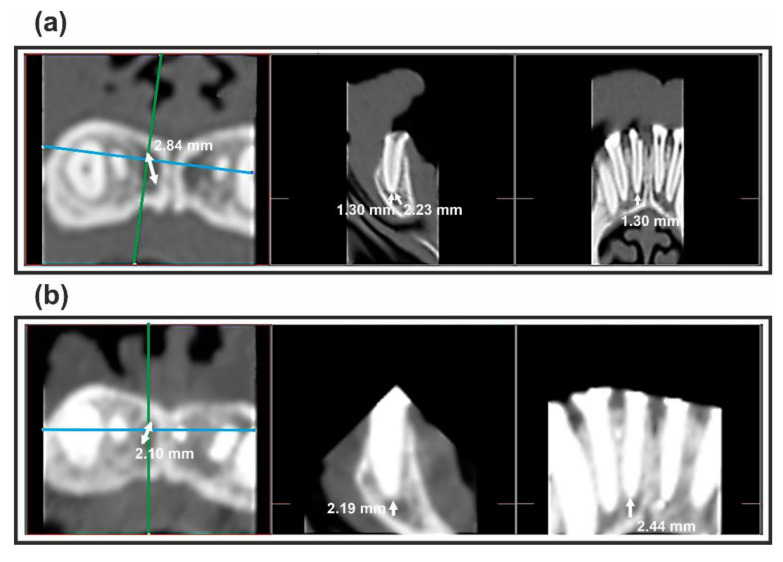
Measurements of the apical lesion of one of the teeth on the axial, sagittal, and transverse planes: (a) The pre-mortem computerized axial tomography (CAT) and (b) the postmortem cone beam computed tomography (CBCT), through the 3D implant planning software.
